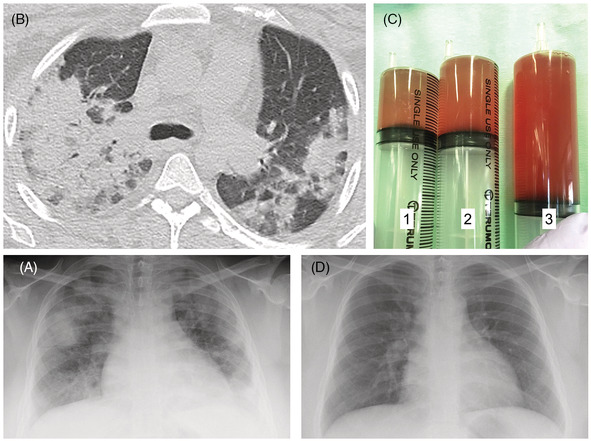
A chest radiograph showing an enlarged heart shadow and bilateral diffuse infiltrates with geographic distribution (A). Computed tomography shows multifocal diffuse consolidation and ground‐glass opacities predominantly in the upper lobes of the bilateral lungs (B). The red colour of the bronchoalveolar lavage fluid from the right upper lobe has intensified with each washing (C). After treatment with steroid therapy, a chest radiograph shows significant improvement (D). BAL, bronchoalveolar lavage
